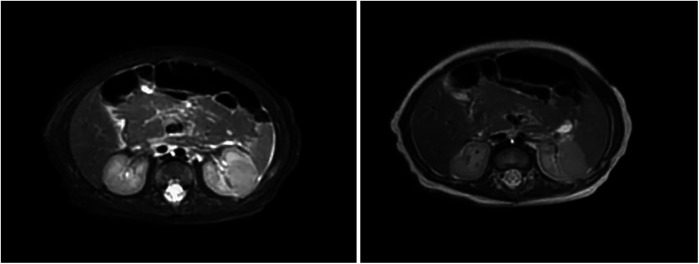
MRI images of the kidney. A well-circumscribed space-occupying lesion in the left kidney [isointense on T1-weighted imaging (T1WI), isointense on T2-weighted imaging (T2WI), slightly hyperintense on diffusion-weighted imaging (DWI), measuring approximately 1.7 × 1.8 × 2.1 cm] demonstrates heterogeneous enhancement. The renal calyces course along its superior margin, with no significant perilesional edema observed.
